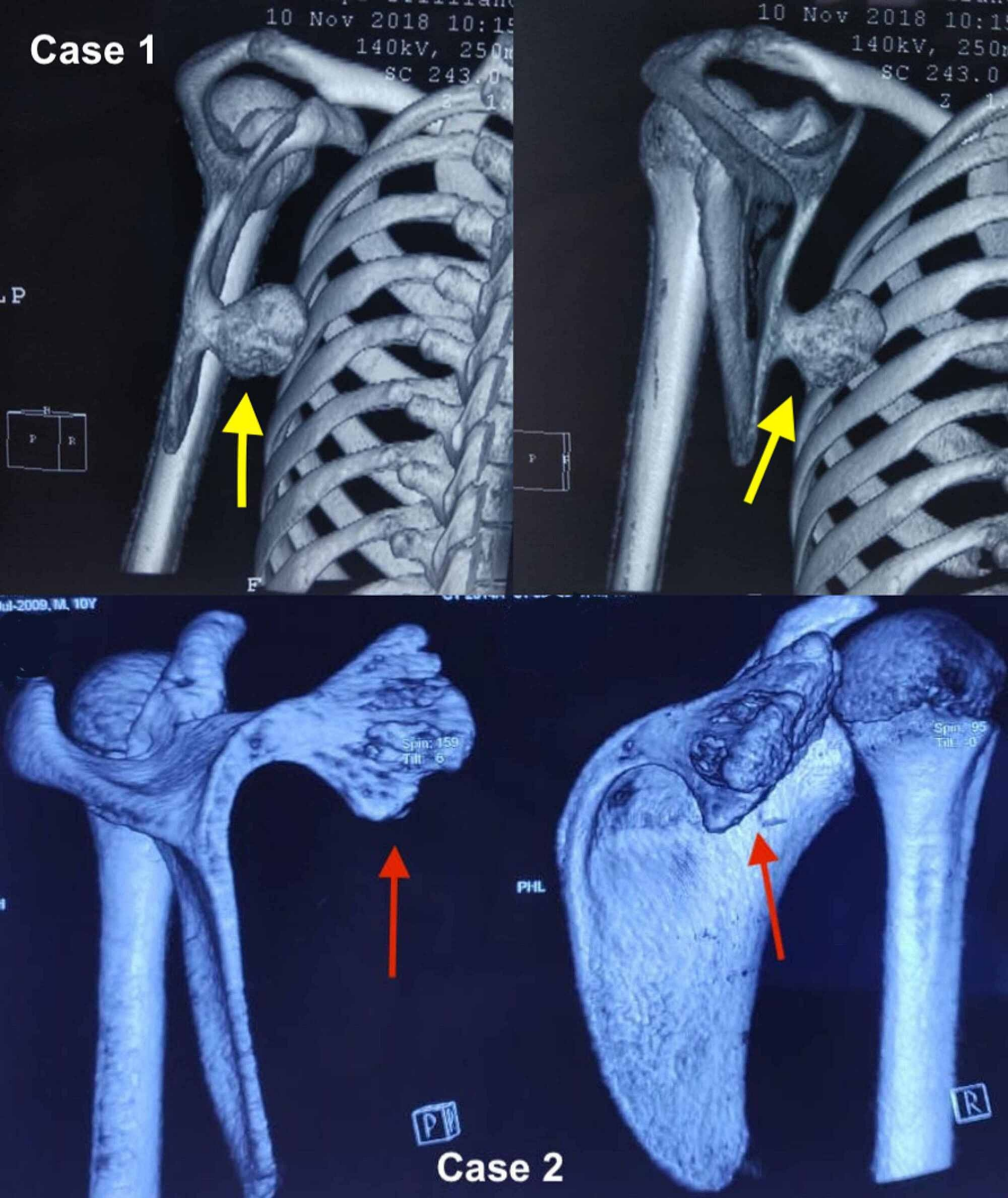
CT scan showing the pedunculated osteochondroma lifting the medial edge of scapula off the thoracic wall in case 1 (yellow arrows) and case 2 (red arrows).
